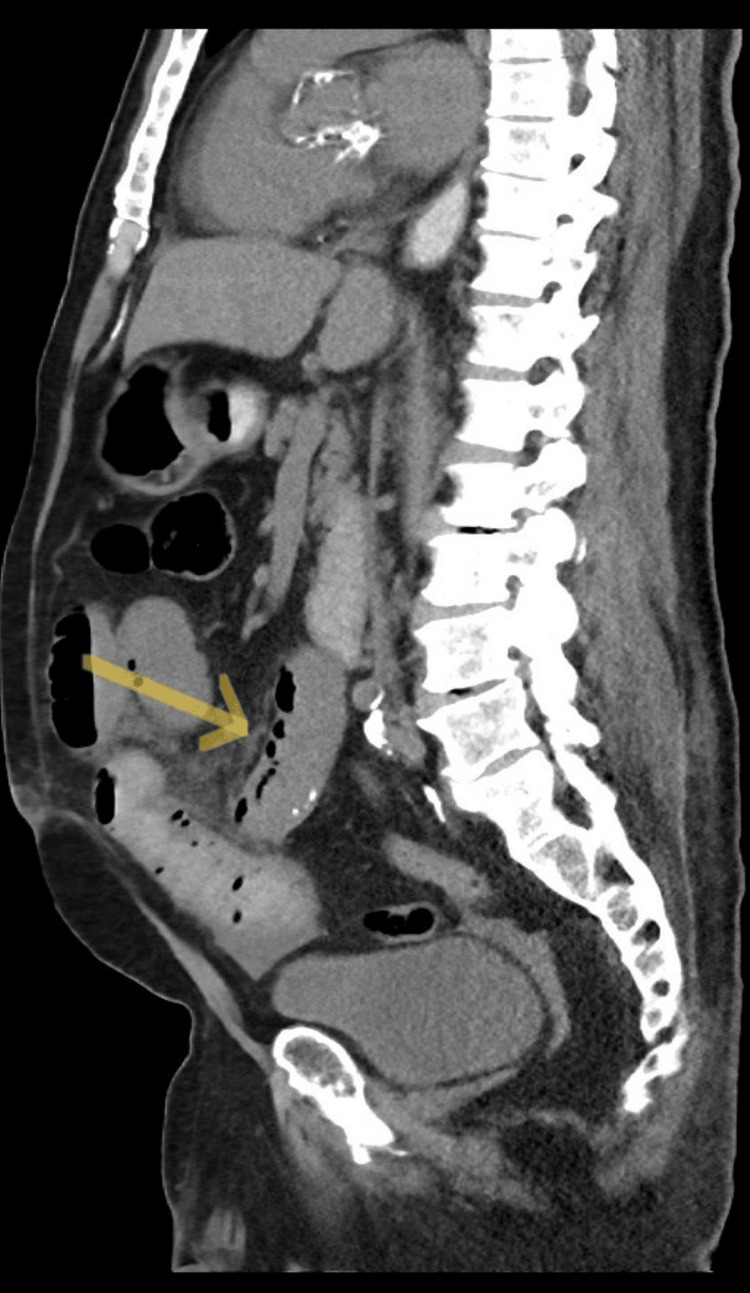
CT scan shows evidence of pneumatosis (see arrow) in portions of bowel ultimately resected.
